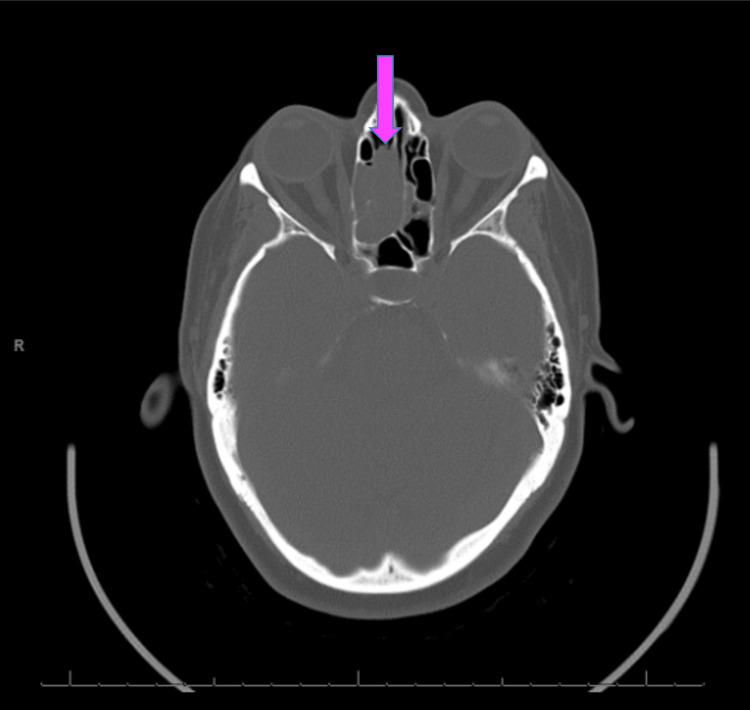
CT scan of the brain revealed a 3.1 × 2.0 × 1.4 centimeter enhancing mass in the superior nasal cavity
